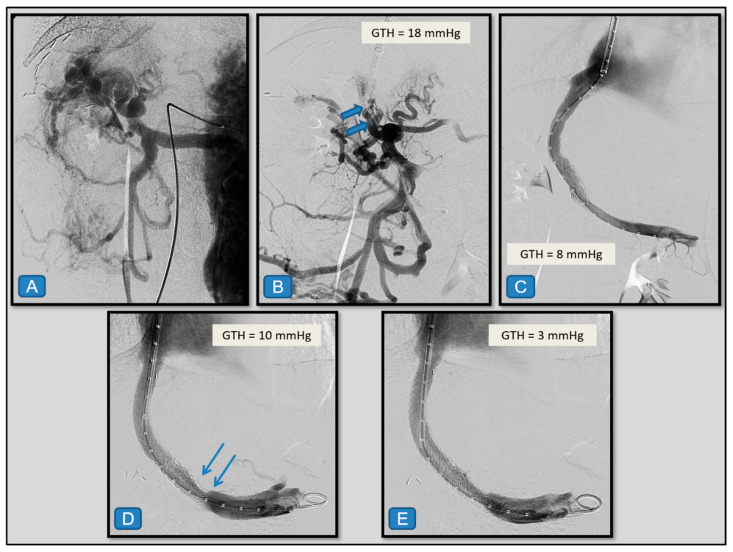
Six-year-old girl with a liver transplant due to extrahepatic biliary atresia, presenting with portal hypertension and bleeding from esophageal varices. (A) Indirect portography shows chronic portal thrombosis with cavernomatosis. (B) Through the right internal jugular vein, a standard ultrasound-guided access was performed. A 10 F introducer sheath was placed in the right atrium, and an angled catheter was used to select the middle hepatic vein and advance the introducer. From this position, multiple punctures were directed toward a large-caliber collateral vein of the cavernomatosis (blue arrows), yielding a transhepatic gradient (GTH) of 18 mmHg. (C) Using a hydrophilic guidewire, this collateral vein was catheterized, and a TIPS was created and dilated with a conventional 8 mm balloon, achieving a final GTH of 8 mmHg. (D) Three years after placement, follow-up imaging demonstrated a patent TIPS with stenosis in its distal segment (blue arrows), with a measured gradient of 10 mmHg. (E) Angioplasty was performed with an 8 mm balloon through a 7 F introducer, followed by placement of a coaxial 8 mm stent, resulting in a final GTH of 3 mmHg.
